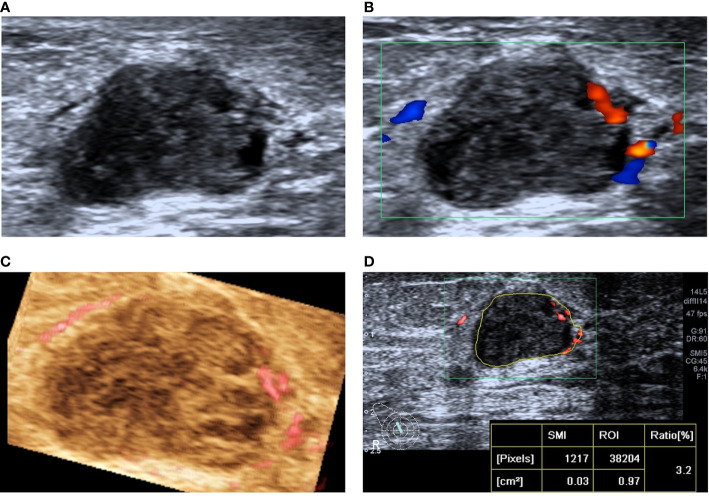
A 78-year-old woman with intraductal papilloma was downgraded from BI-RADS 4A to BI-RADS 3 after SMI assessment. (A) Two-dimensional ultrasound shows an irregular hypoechoic lesion. (B) CDFI shows the striated blood flow signal around the lesion. (C) SMI shows a dotted and linear VA. (D) The vascular index was measured by delineating the lesion edge on the plane with the most abundant blood vessels, and the value was 3.2.
